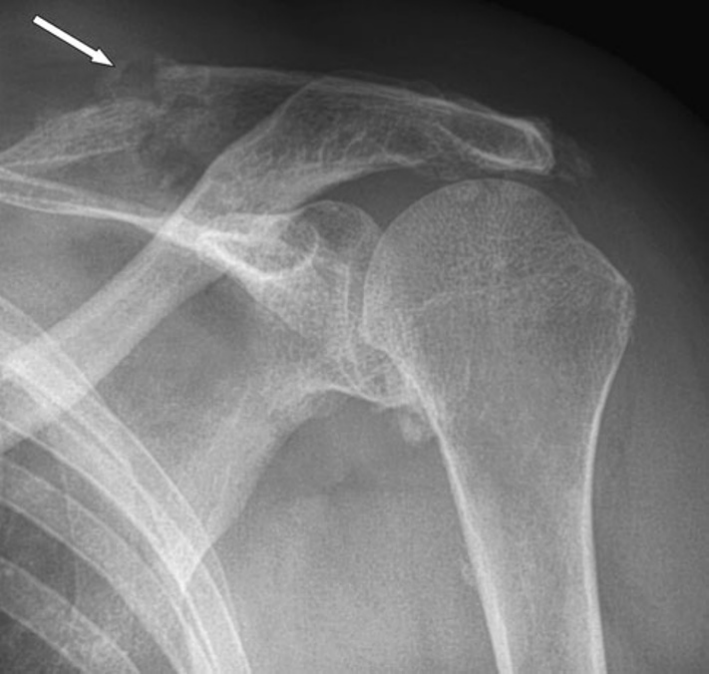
(Patient 1) X-ray of left shoulder showing scapular spine fracture, two months after initial symptoms (*white arrow*)
